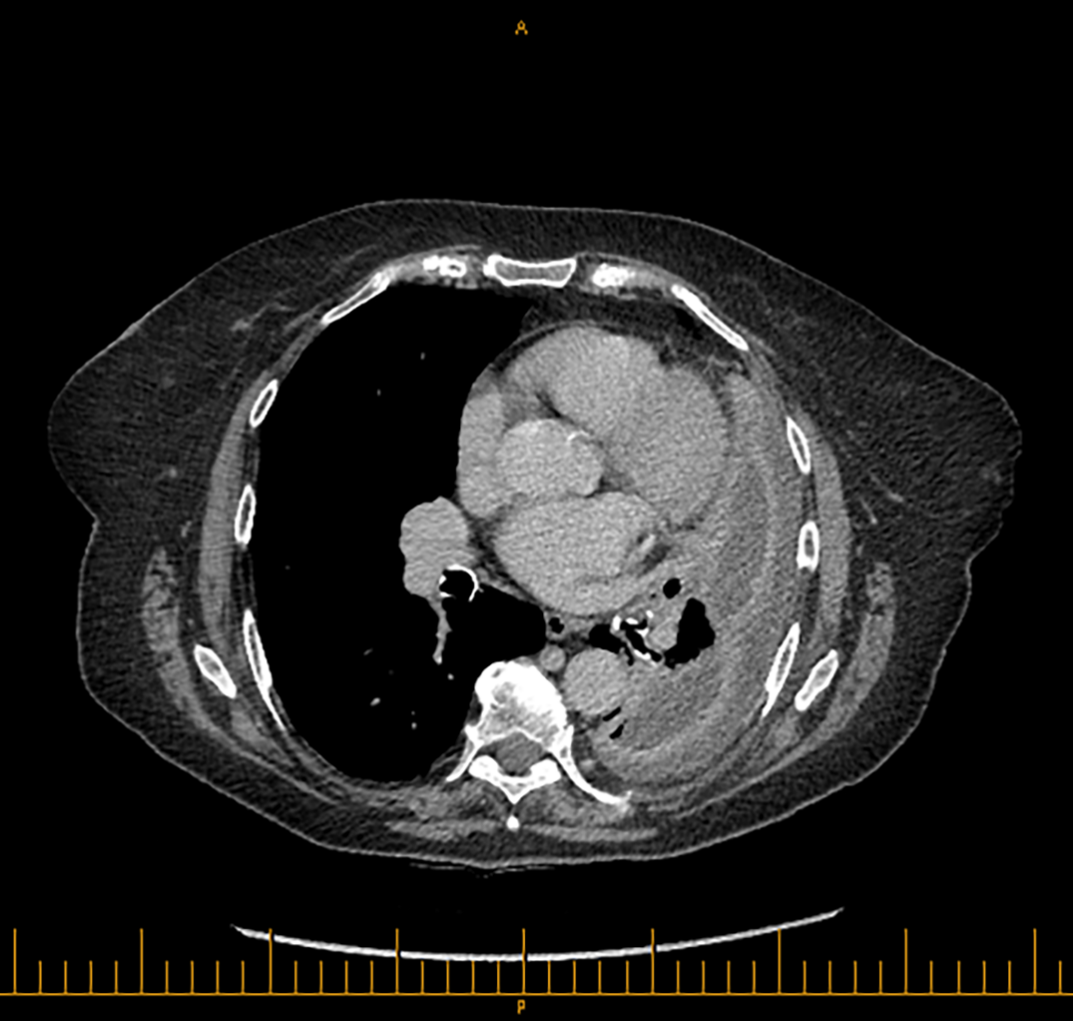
Axial slice of chest computed tomography scan taken 2 months following admission.
